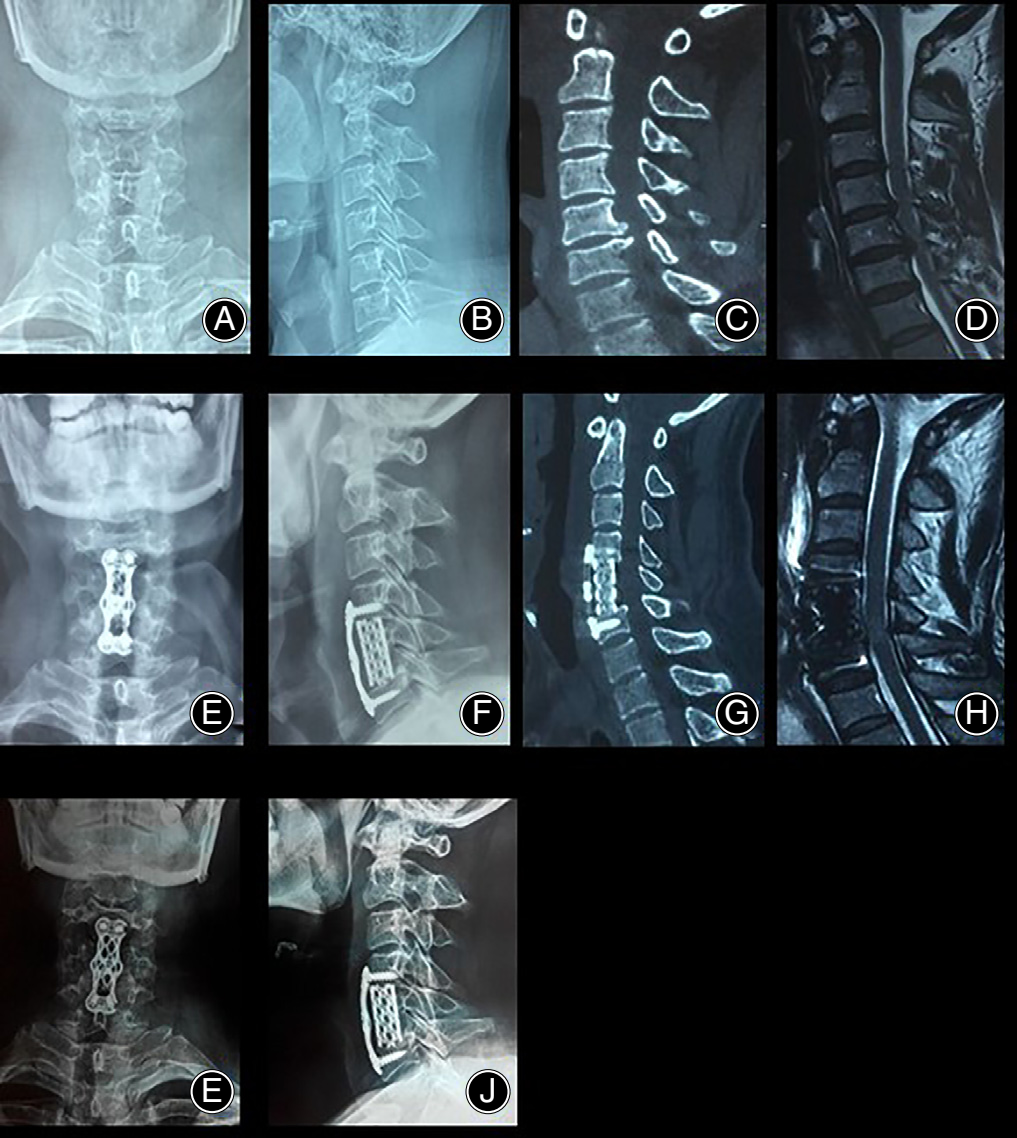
A representative case. Male patient, 56 years old, with numb hands and weakness in both lower limbs for 2 years, exacerbated by 1 month. (A and B) Preoperative x‐ray report of cervical curvature straightening. (C) CT report of osteophyte formation in the posterior margin of 5–6 segments of cervical spine. (D) Preoperative MRI showed that the anterior compression of 5–6 cervical spinal cord was obvious. (E and F) After ACCF operation, titanium mesh and titanium plate were fixed firmly. (G) Postoperative CT showed that the posterior edge of cervical vertebrae 5–6 had been removed. (H) Postoperative MRI showed that the compression of cervical spinal cord at 5–6 segments had been relieved, and there was no obvious compression of spinal cord. (I and J) There was no obvious subsidence of titanium mesh X‐rays 3 months after surgery.
